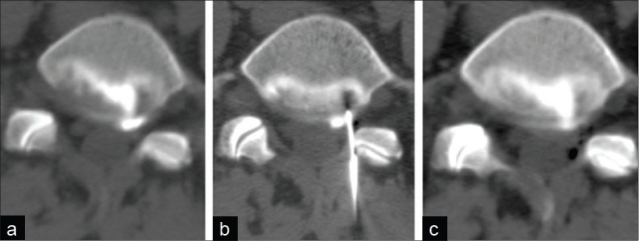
Transaxial computed tomography (CT) scan at the L5-S1 level. Before the CT-guided aspiration, the discal cyst is hyperdense (a). After CT-guided needle placement (b), the contents of the cyst were aspirated. Subsequently, the cyst is substantially smaller (c)
